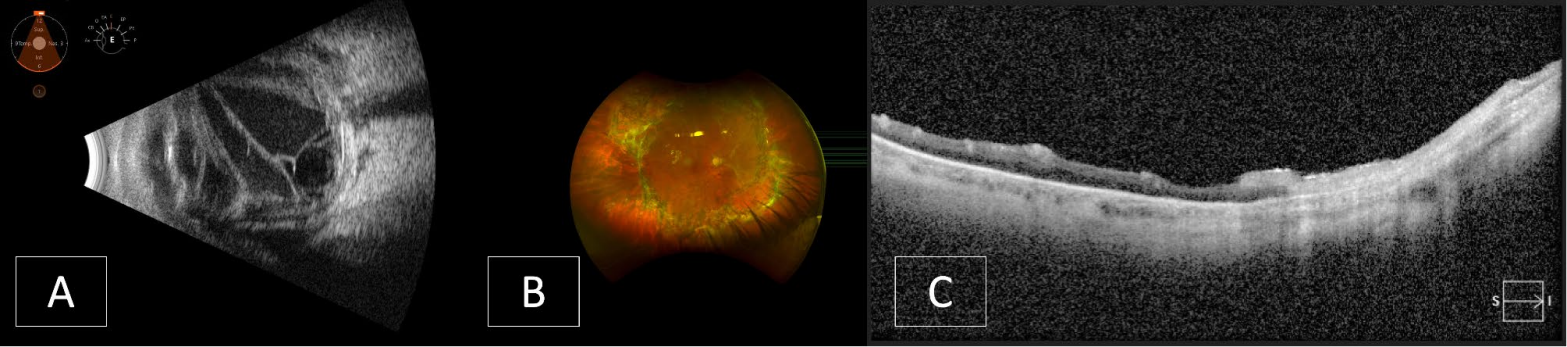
Case 2 images. A: B-scan ultrasound at presentation. B: Optos image following the third pars plana vitrectomy. C: Optical coherence tomography 6 months post-trauma
